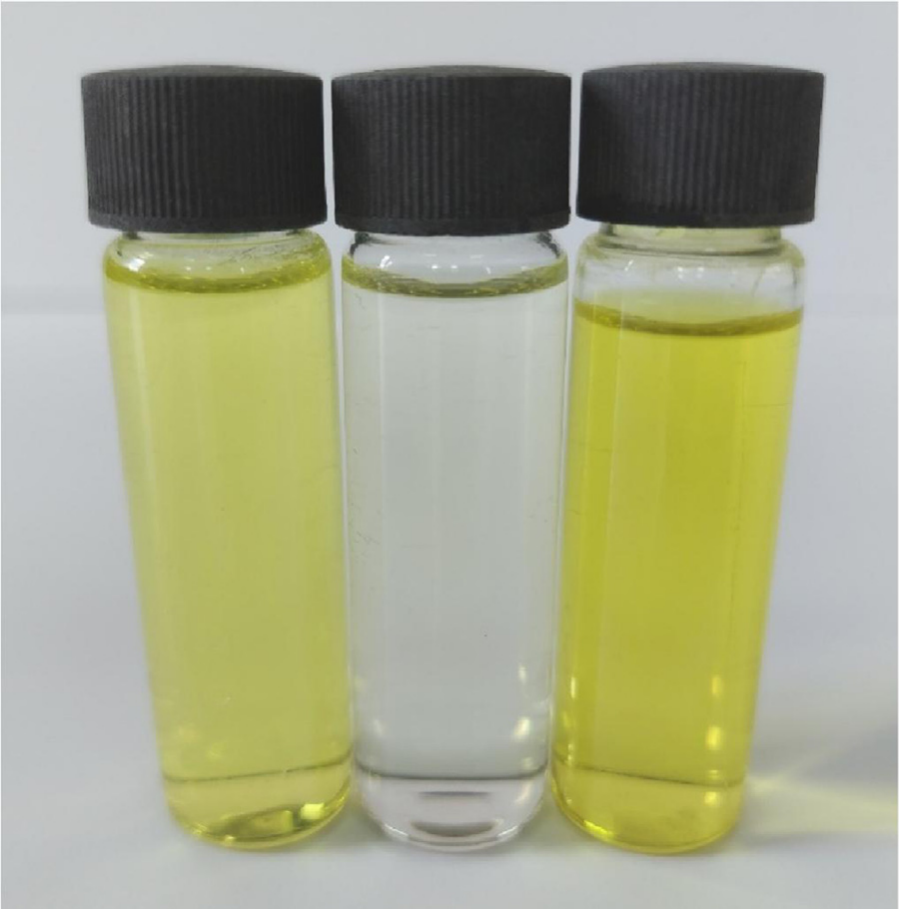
Color change upon mixing PA acceptor (*far left; yellow*) with the donor (*middle; colorless*) to generate the CT complex (*far right; instance yellow*). (For interpretation of the references to color in this figure legend, the reader is referred to the web version of this article.)
